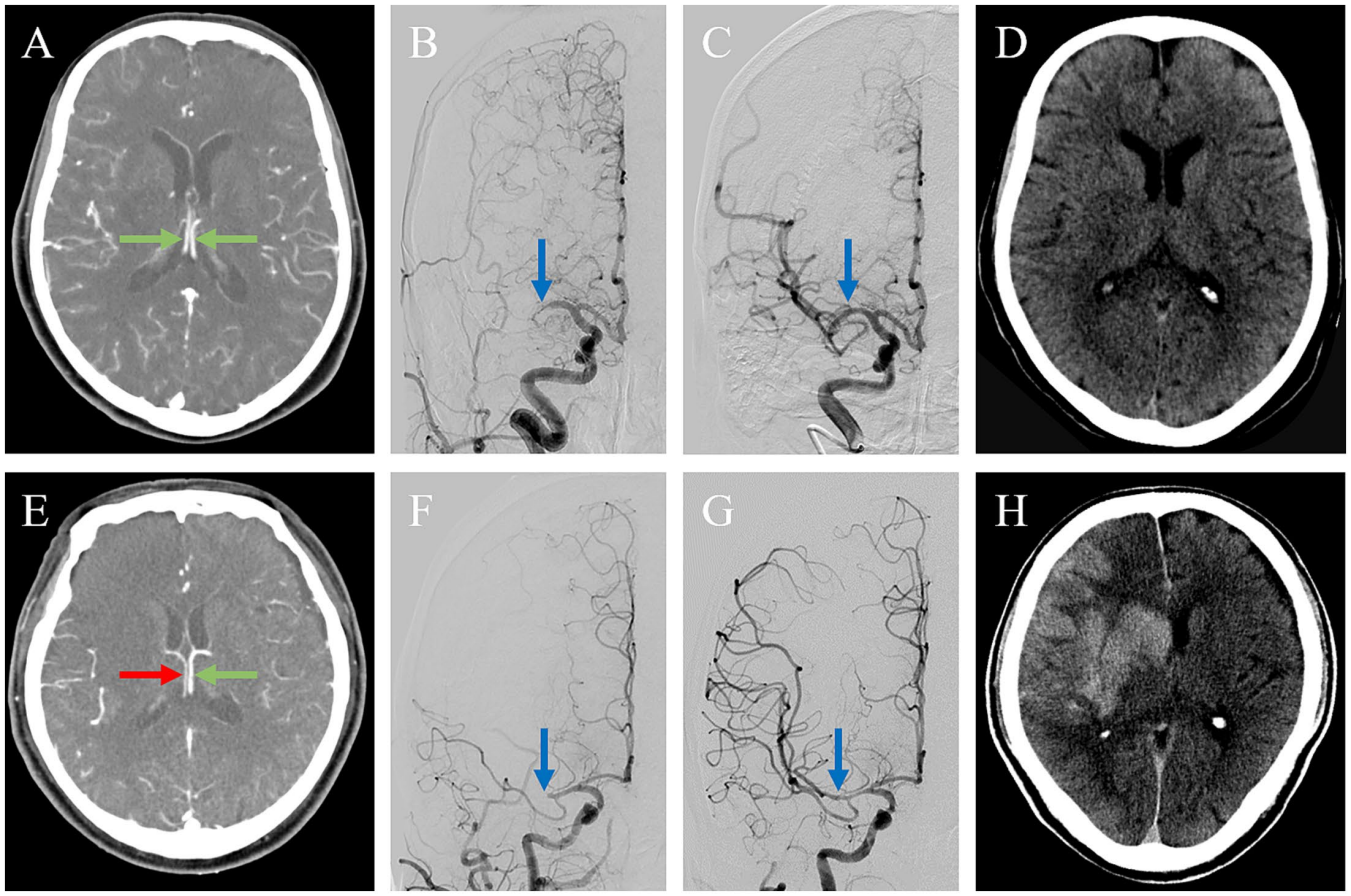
Association between ICV asymmetry and HT. Two patients with acute ischemic stroke due to occlusion of the M1 segment of the right middle cerebral artery (MCA), (A–D) 1 with internal cerebral veins (ICVs) symmetry and (E-H) 1 with ICVs asymmetry. In the first patient, axial maximum intensity projection imaging shows the ICVs running parallel to each other and symmetrical opacification (green arrows) (A). After endovascular thrombectomy, the initial proximal MCA occlusion (blue arrow) (B) was successfully reperfused with a modified Thrombolysis in Cerebral Infarction (mTICI) of 3(blue arrow) (C). The non-contrast CT on 24 h showed a small acute infarct without hemorrhagic transformation. The second patient exhibited a poor ICV profile in the right hemisphere [(E), red arrow] and good opacification in the left (green arrow). The thrombus on the right proximal MCA (blue arrow) (F) was successfully reperfused with an mTICI of 3 (blue arrow) (G). The non-contrast CT on 24 h showed hemorrhagic transformation with a cerebral hernia (H).
